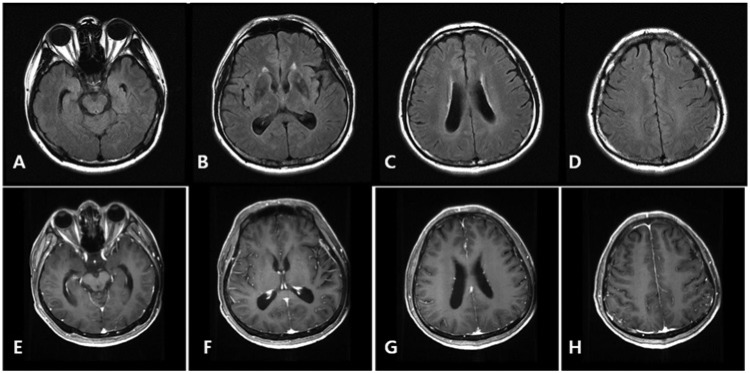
Follow-up axial FLAIR (A-D) and T1WI postcontrast (E-H) imaging show subtle focal hyperintensities without mass effect. No abnormal enhancing lesions are seen in parenchymes but asymmetric focal prominent leptomeningeal enhancement in right parietal cortex is noted.
